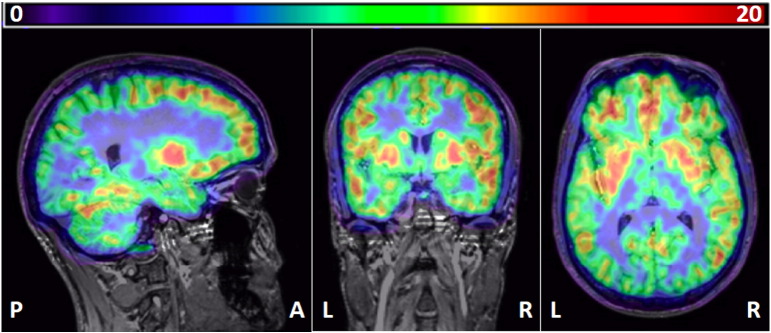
Example of a parametric map, co-registered onto the subject's MRI scan. Left, sagittal; middle, coronal; right, transverse. Note high binding in the putamen and pallidum and intermediate binding in the hippocampus and thalamus. Colour bar: *V*_T_ values. P, posterior; A, anterior; R, right; L, left.
